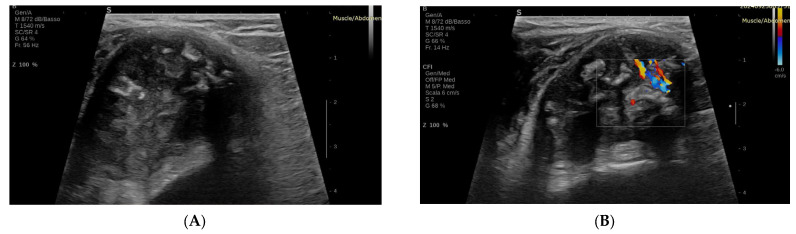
Conventional US of a benign STT (Chondroma) (A): B-mode US image; (B): color Doppler ultrasound image.
